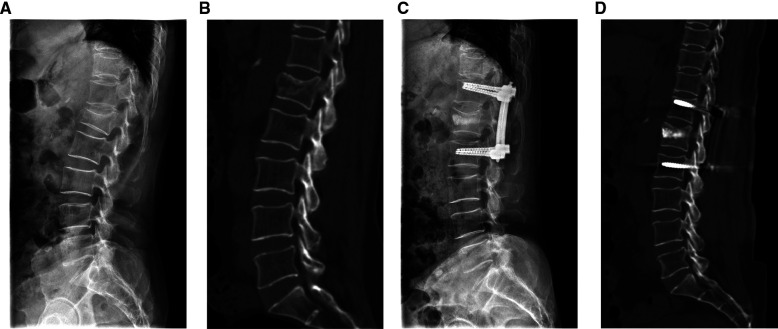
A patient treated with PPSR. (A,B) The preoperative sagittal radiograph (A) and CT image (B). (C,D) The postoperative sagittal radiograph (C) and CT image (D).
